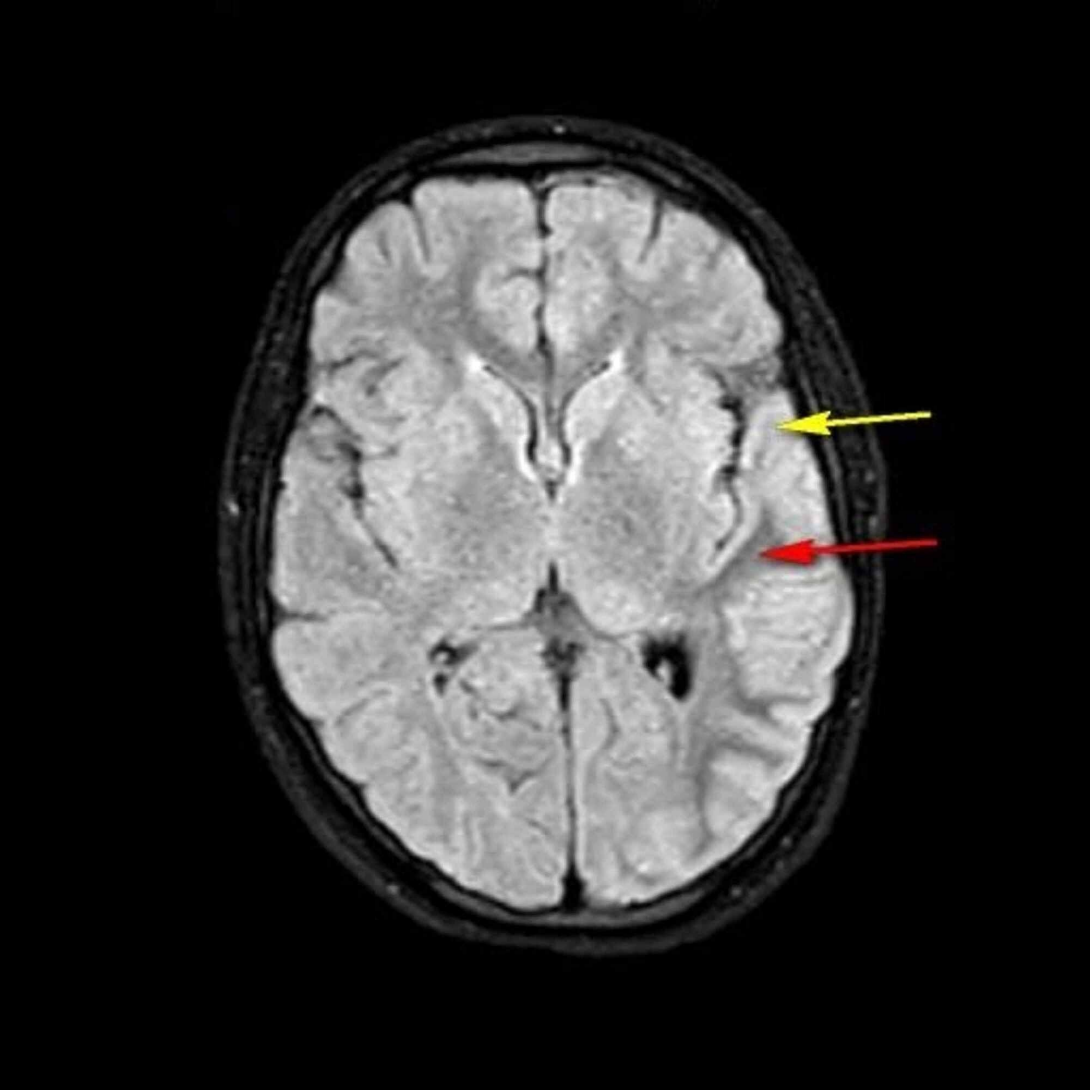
MRI of the brain: T2-FLAIR sequence The T2-FLAIR image of the patient is shown. The red arrow indicates subcortical hypointensity, and the yellow arrow points to the overlying cortical hyperintensity, which are the classical MRI findings to be expected in NKH-induced seizures. NKH, nonketotic hyperglycemia; T2-FLAIR, T2-weighted fluid-attenuated inversion recovery
